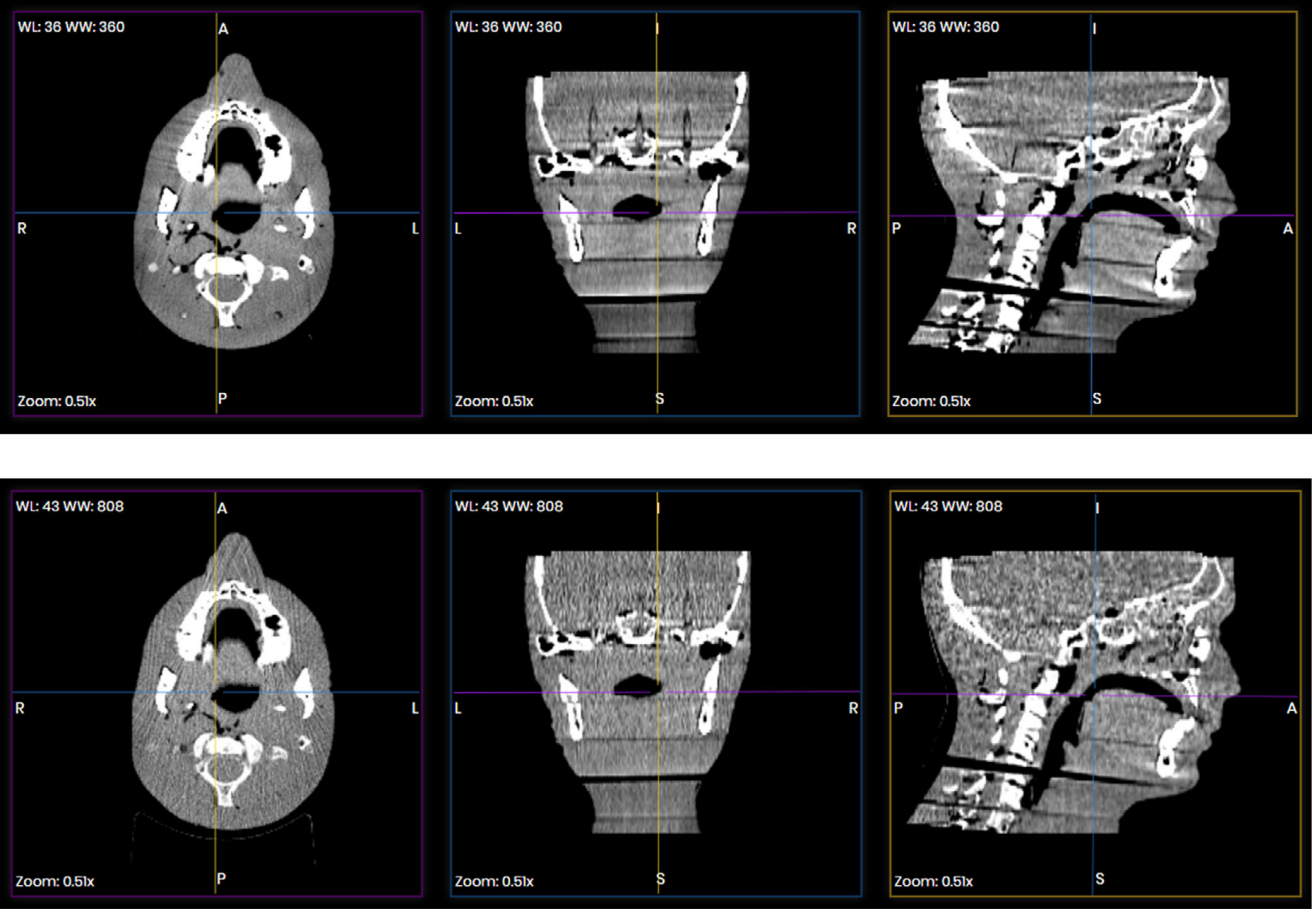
Upper panel shows full‐dose Head and Neck CBCT (100 kVp, 150 mAs, 15 fps), lower panel shows the low‐dose CBCT (80 kVp, 50 mAs, 7 fps), both registered to the planning CT.
